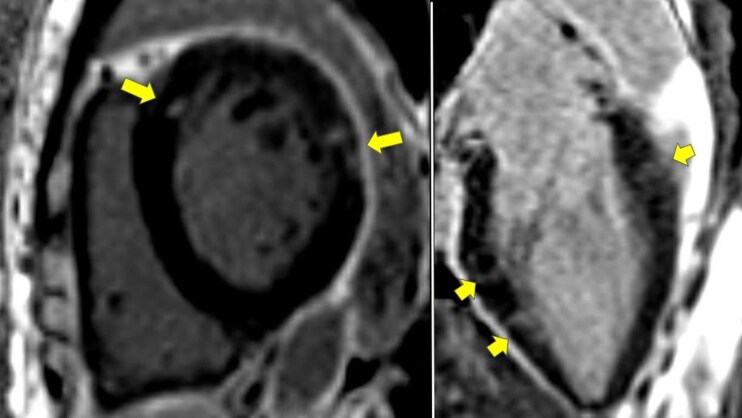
Cardiac magnetic resonance imaging showed mid-wall late gadolinium enhancement at the anteroseptal and anterolateral segments and subepicardial late gadolinium enhancement at the basal lateral segment which signify for non-ischaemic LGE (yellow arrow).
